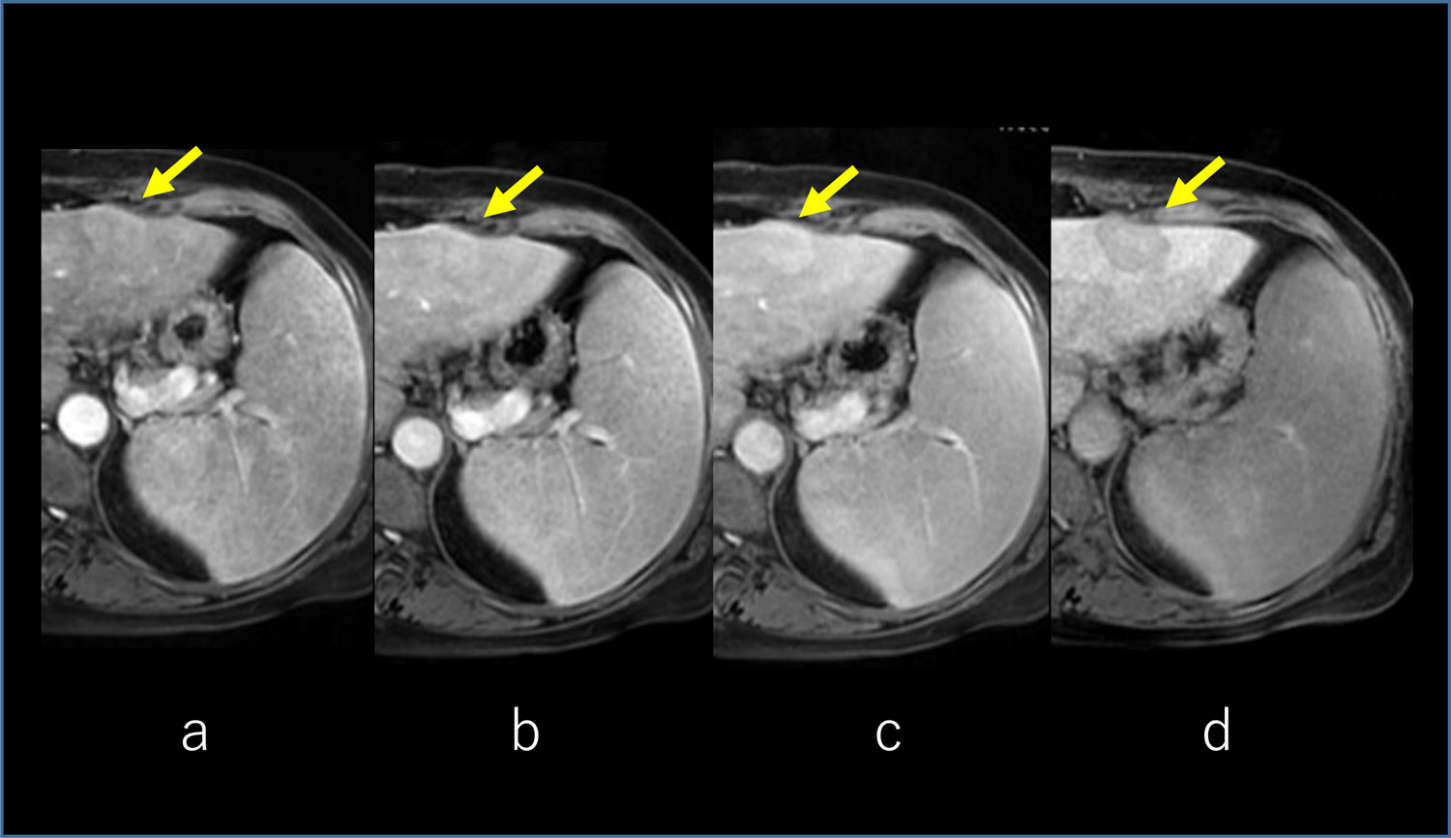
Gd-EOB-DTPA-enhanced MRI. Arterial, portal, and transitional phases of the dynamic scan show enhancement of the tumor with central part sparing (arrow) (A, B, C). The hepatobiliary phase shows a faint uptake of contrast in the central part of the tumor (arrow) (D). MRI, magnetic resonance imaging.
